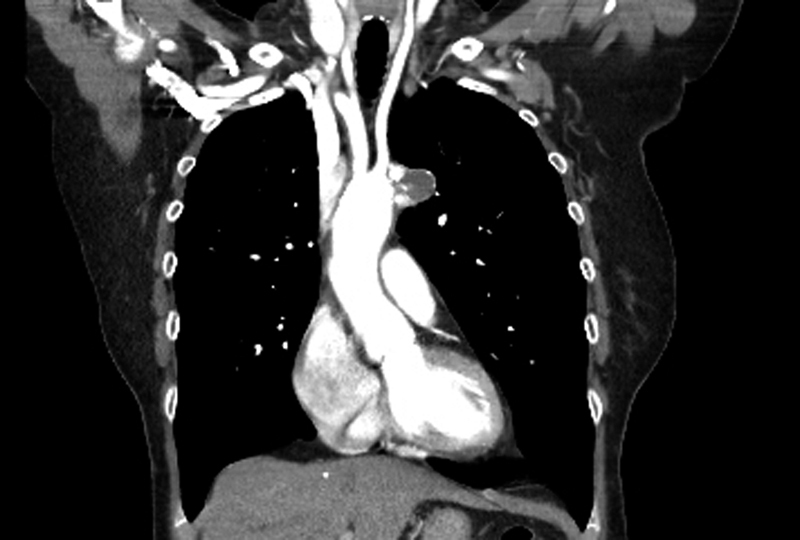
Follow-up computed tomography (CT) aorta showing no contrast filling into the aneurysm.
